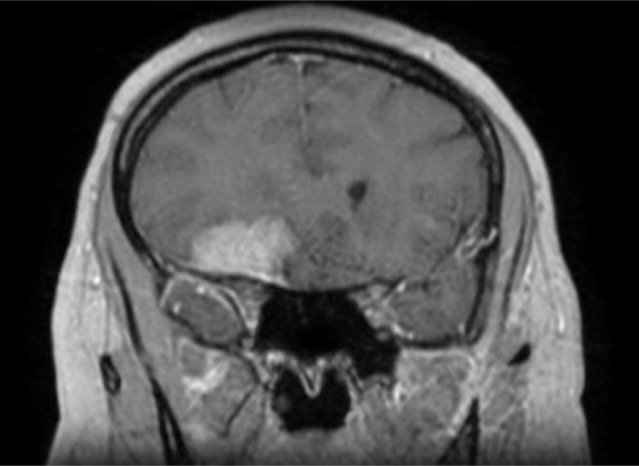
Gadolinium-enhanced T1-weighted MRI scan, coronal section, showing a large extra-axial tumor inserted on the dura mater of the right sphenoid wing, with the specific dural tail sign, suggestive of sphenoid wing meningioma.
